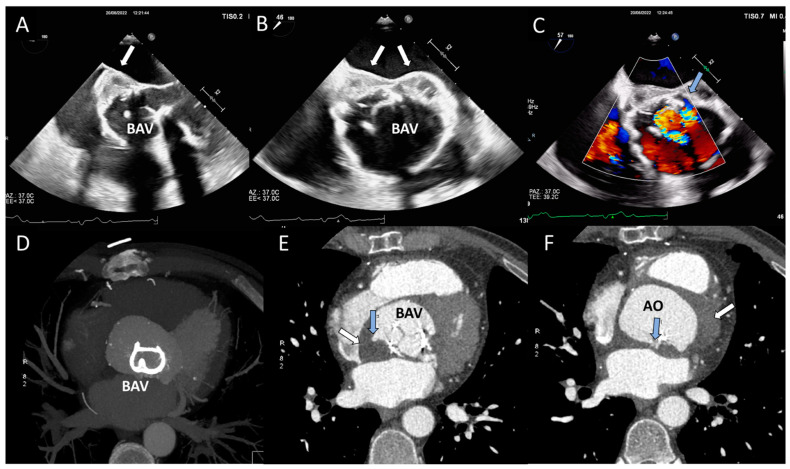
Periprosthetic abscess and detachment in a patient with bioprosthetic aortic valve. (A,B) Transesophageal echocardiogram in mid-esophageal view showing iso-hypoechoic space posteriorly to the bioprosthetic aortic valve (BAV). (C) Transesophageal echocardiogram in mid-esophageal view, with color Doppler showing paravalvular posterior leak and regurgitation (light blue arrow). (D) Contrast CT 3D volume rendering: The struts of the BAV are hyperdense and clearly visible. (E,F) The abscess (white arrow) appears like an isodense region posterior to the BAV; the light blue arrow indicates the presence of multiple leaks with contrast inside.
